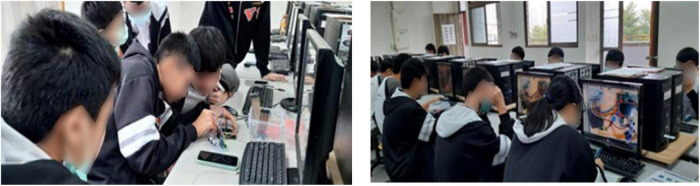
The practical operations and stimulating applications of the ultrasonic sensor.
